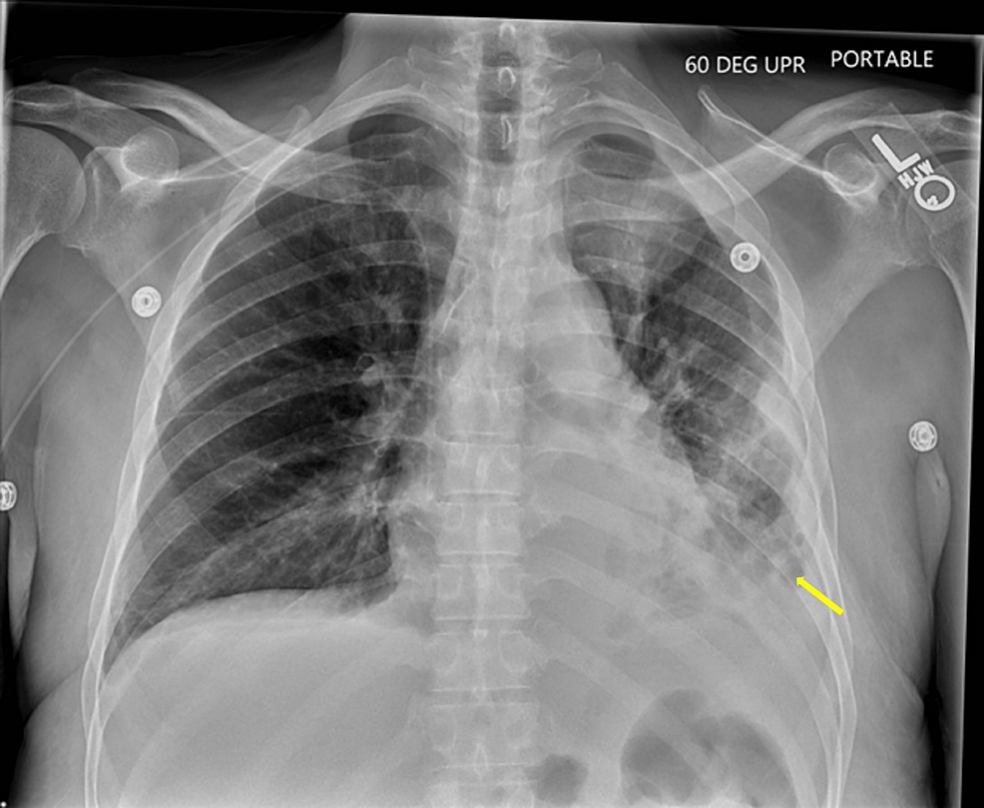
Chest X-ray demonstrating the stable left-sided pleural effusion relatively unchanged during admission
